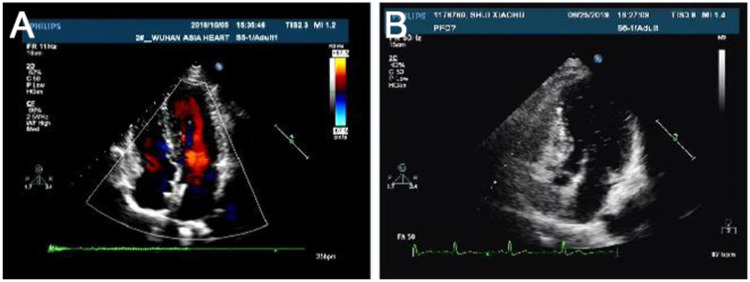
(A) Patient's admission echocardiogram; (B) patient's follow-up echocardiogram one year later.
